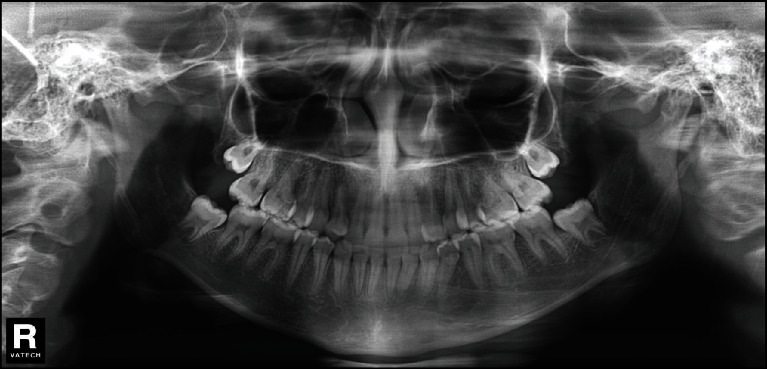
Posttreatment panoramic radiographs.
